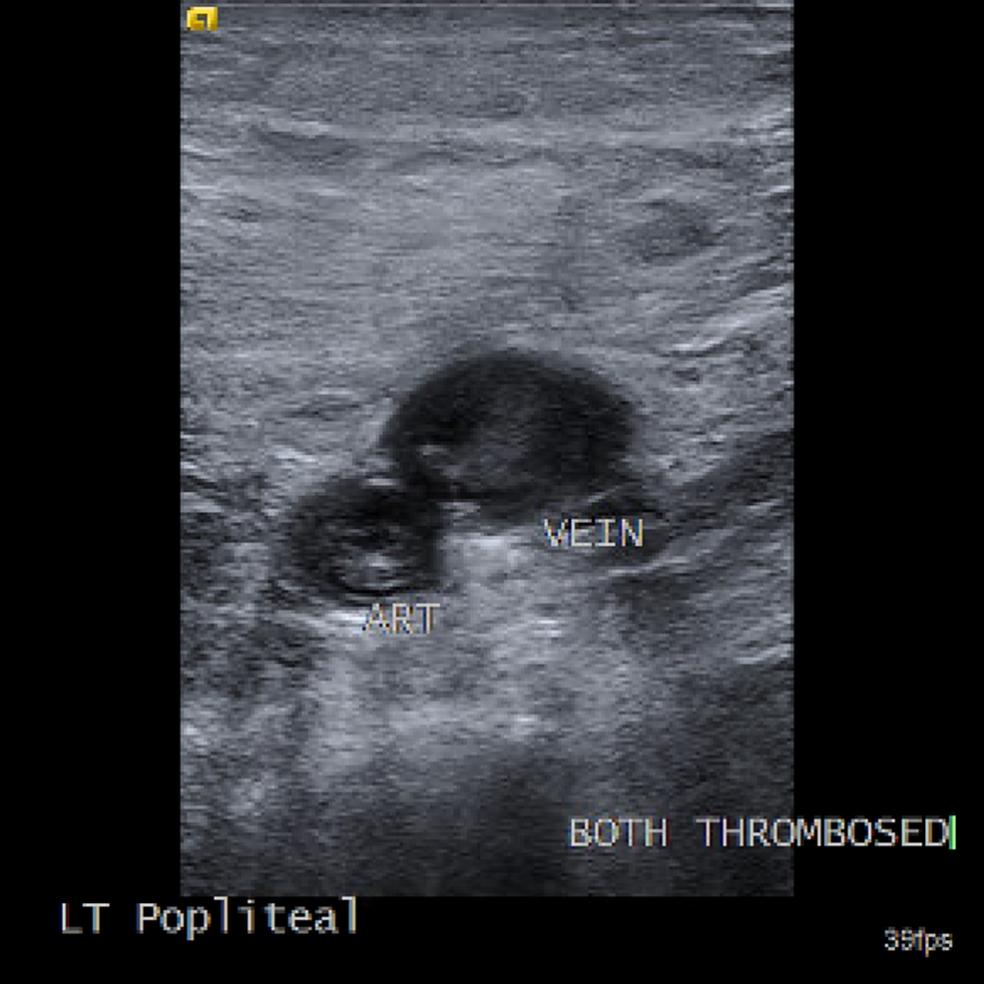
Left popliteal artery and vein, visualized in the axial view of ultrasound, are both thrombosed.
